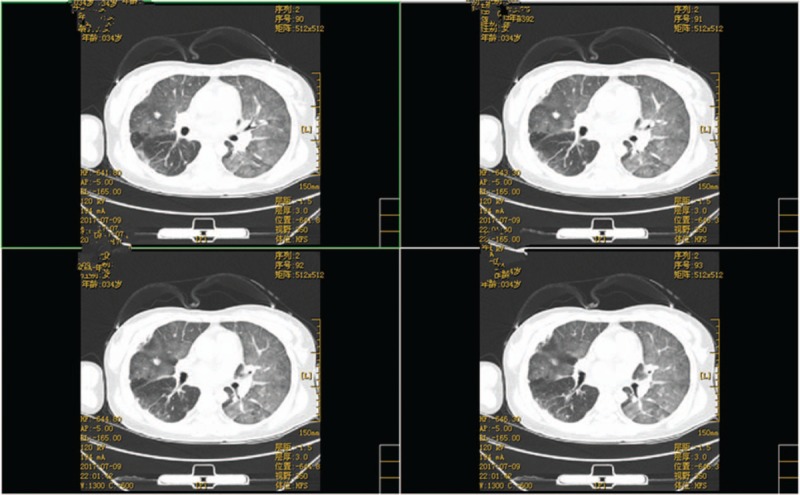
Preoperative CT image. Chest CT revealed pulmonary congestion. CT = computed tomography.
